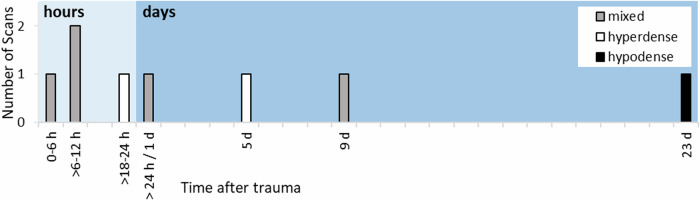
Densities of SDCs at different times after trauma observed in CT
